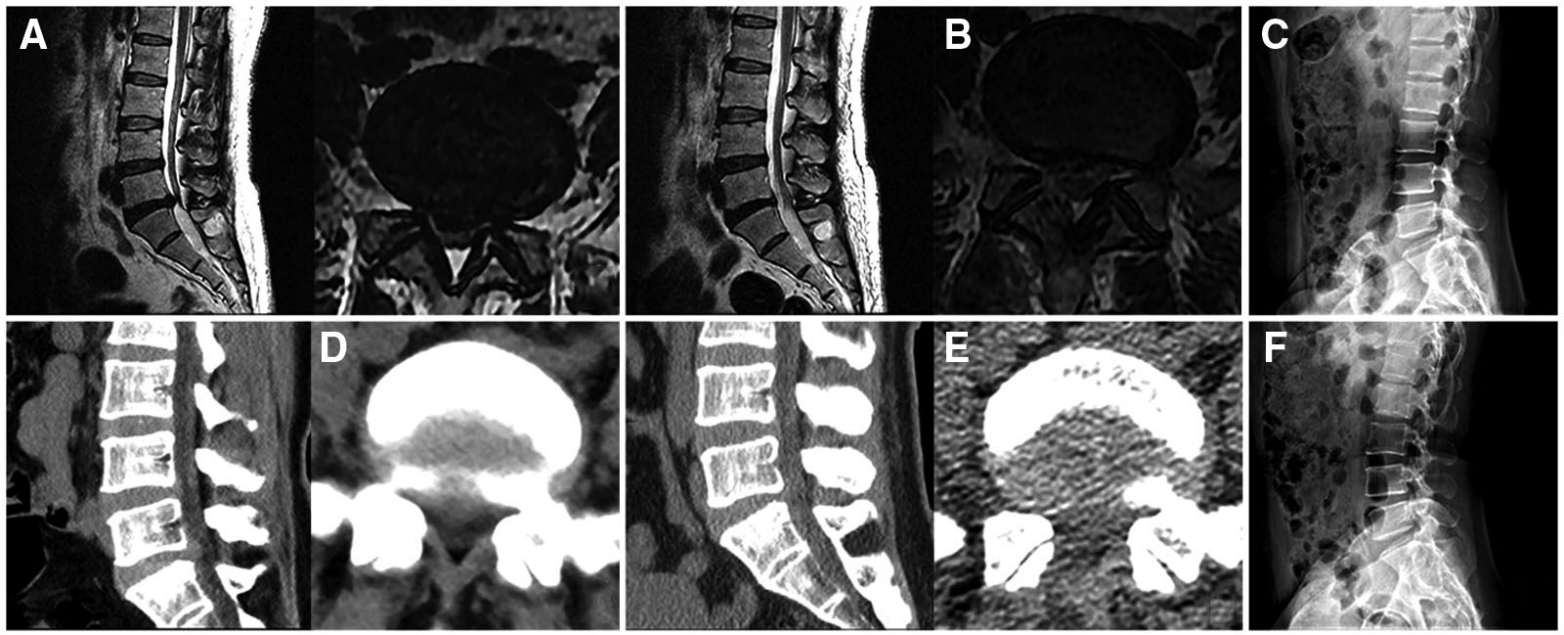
Images of a patient who underwent percutaneous endoscopic lumbar discectomy *via* the interlaminar approach. (A) A right L5–S1 disc herniation is shown on preoperative magnetic resonance imaging. (B) Postoperative imaging shows removal of the herniated disc, preservation of the annulus fibrosus, and a defect in the ligamentum flavum. (C) Preoperative lateral plain radiography of the lumbar spine. (D) L5–S1 disc herniation on preoperative computed tomography. (E) A window in the lamina and defects in the ligamentum flavum are seen after surgery. (F) Postoperative lateral plain radiography for the lumbar spine.
